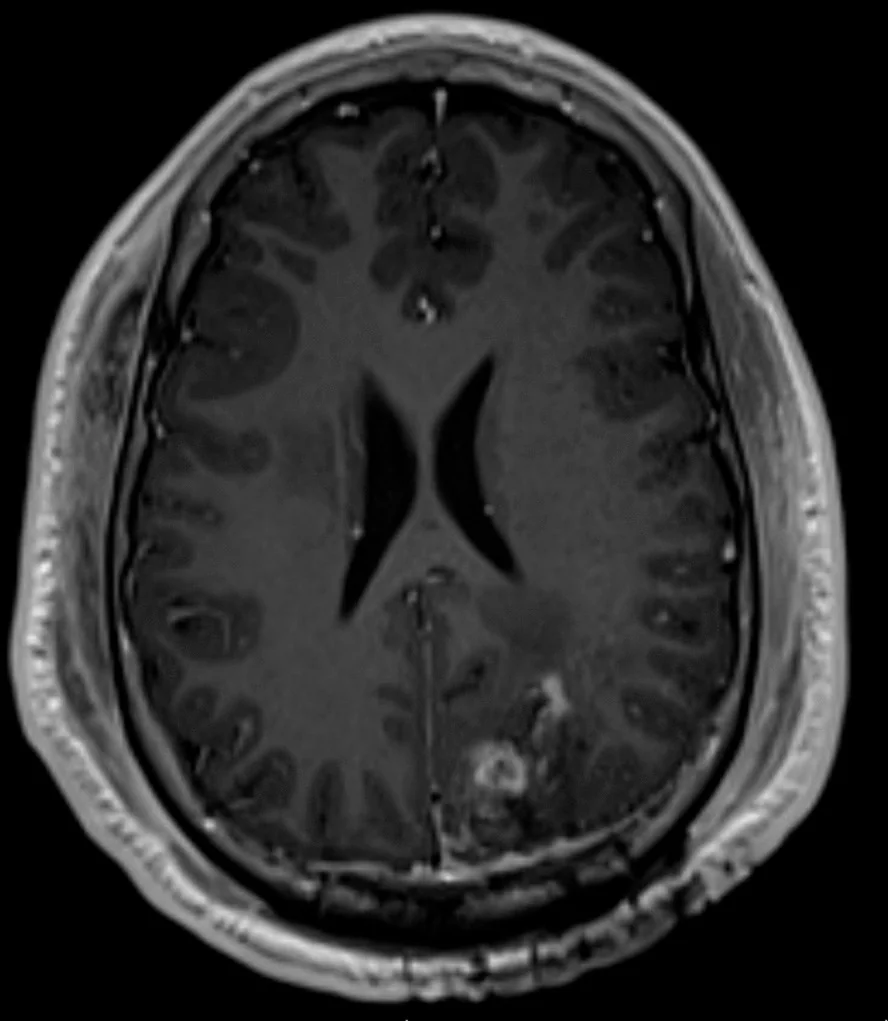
MRI brain T1 post-contrast sequence following resection of the parieto-occipital lesion, revealing gross-total resection of the lesion with some enhancing surgical products visible
